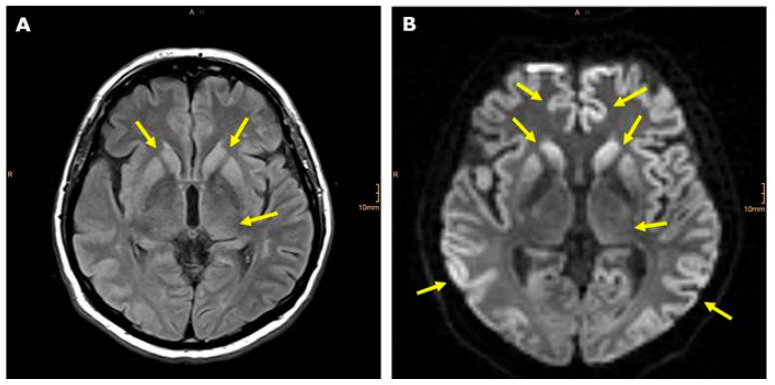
(A) Axial Fluid-attenuated inversion recovery (FLAIR)-weighted magnetic resonance imaging (MRI) shows hyper-intense basal ganglia and left thalamus. (B) Diffusion-weighted imaging (DWI) revealed a restriction of diffusion within the bitemporo-parietal and bifrontal region of the cerebral cortex, and again within basal ganglia and left thalamus (yellow arrows).
